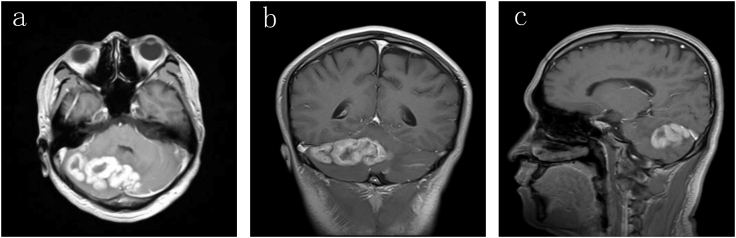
Magnetic resonance imaging showing cerebellar mass lesions within the bilateral cerebellar hemispheres with strong enhancement.
